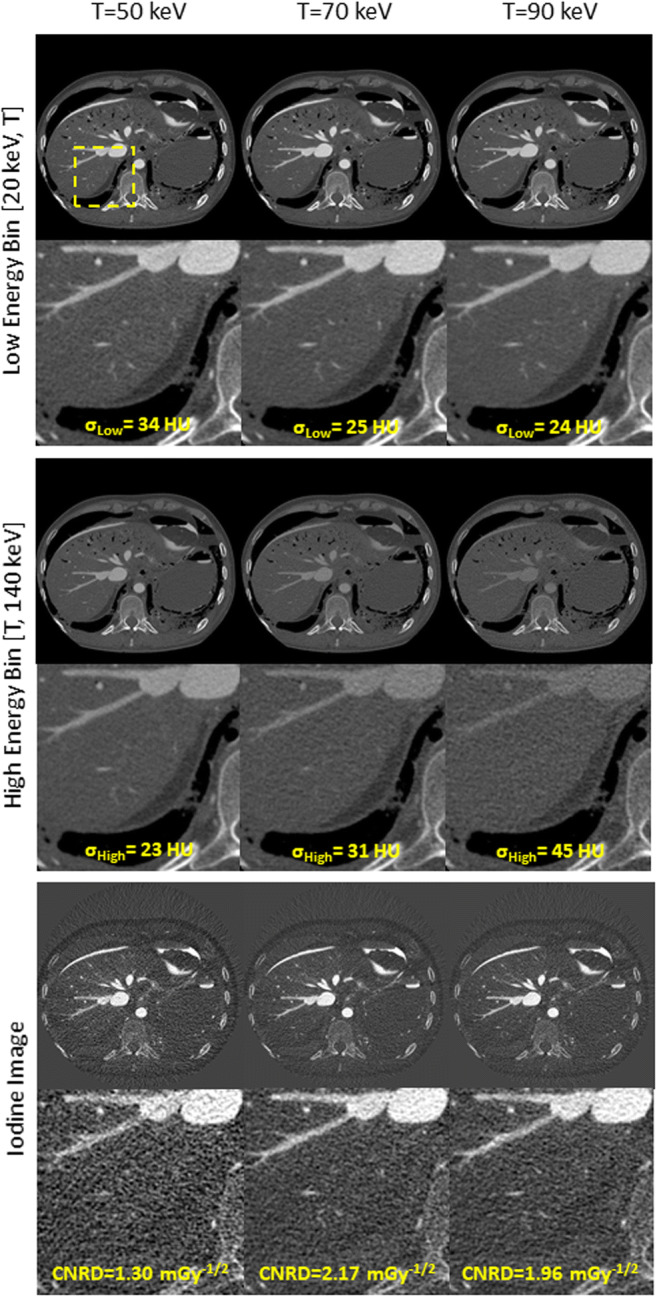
Post-mortem CT angiography using a tube voltage of 140 kV for thresholds of 50 keV (left column), 70 keV (middle column), and 90 keV (right column). Low energy bins (top row), high energy bins (middle row), and iodine maps (bottom row) (bin images *C* = 300 HU, *W* = 1400 HU; material maps *C* = 15 mg/mL, *W* = 30 mg/mL)
